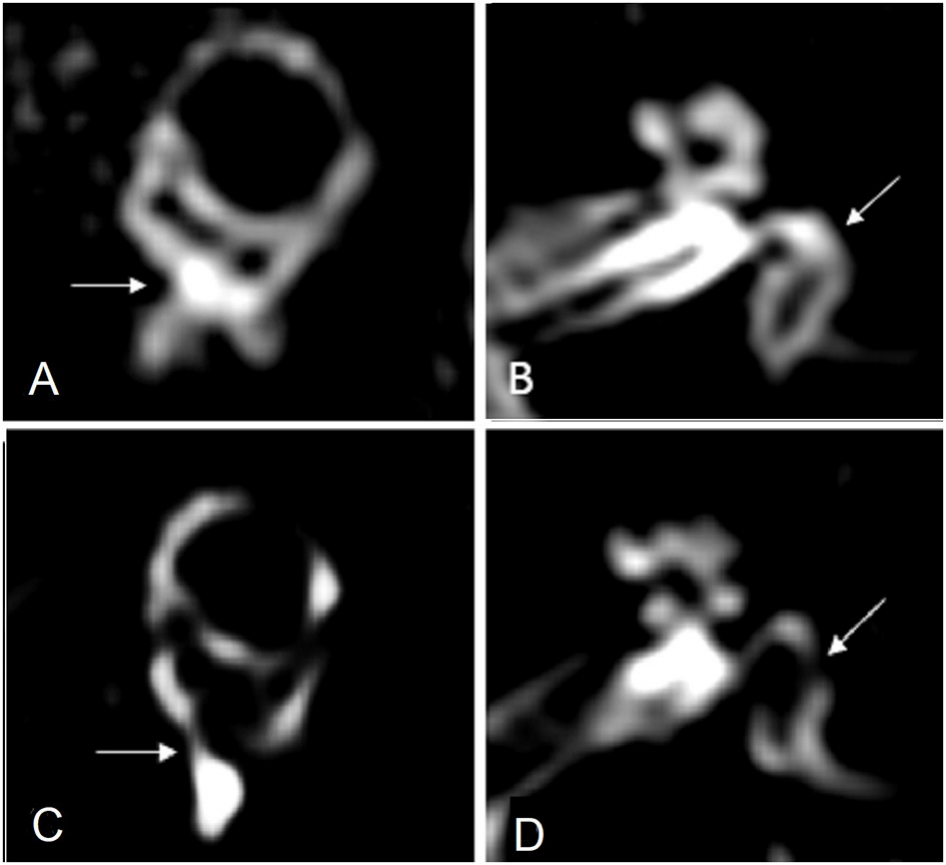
(A) MR imaging oblique sagittal reconstruction parallel to the superior semicircular canal of a healthy ear shows superiorly the vestibular endolymphatic space and inferiorly the perilymph filling the inferior third of the vestibule with preservation of the perilymph signal medial to the oval window (arrow). (B) MR imaging axial reconstruction parallel to the lateral semicircular canal at the inferior third of the vestibule in a healthy subject, showing the vestibule filled by the perilymph (arrow). (C) MR imaging oblique sagittal reconstruction parallel to the superior semicircular canal in a patient with Menière disease shows enlargement of the vestibular endolymphatic space bulging into the inferior third of the vestibule and contacting the oval window (arrow), with the consequent absence of the normal perilymph signal. (D) MR imaging axial reconstruction parallel to the lateral semicircular canal at the inferior third of the vestibule shows the vestibular endolymphatic space contacting the oval window, “VESCO” (arrow).
